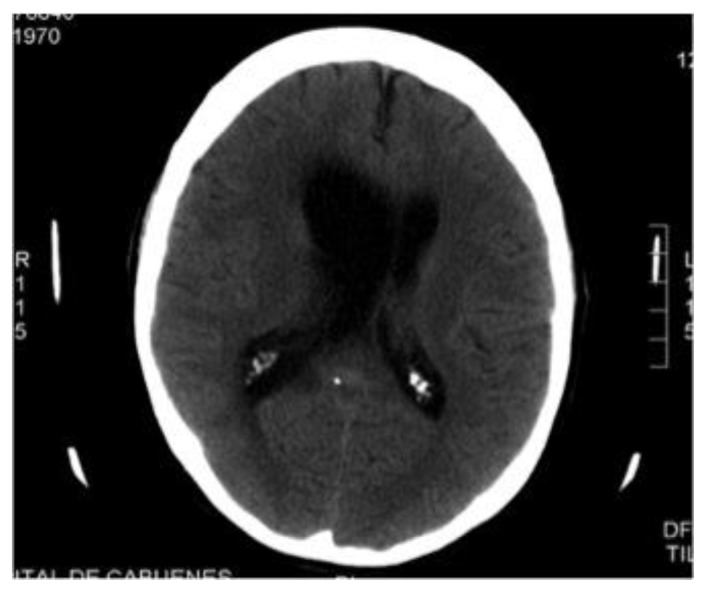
Cranial computed tomography reveals marked hydrocephalus and hipodensity around ventricles.
